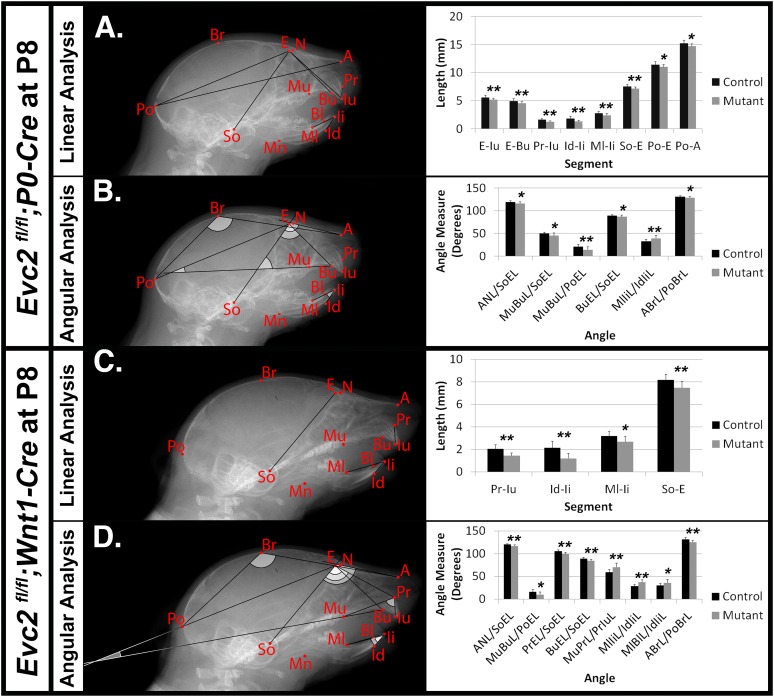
Differential mid-facial defects between *Evc2 P0* mutants and *Evc2 Wnt1* mutants at P8. Lateral X-ray cephalogram indicates the linear (A) and angular (B) measurements with significant differences between controls and *Evc2 P0* mutants. Lateral X-ray cephalogram indicates the linear (C) and angular (D) measurements with significant differences between controls and *Evc2 Wnt1* mutants. Graphs indicate the linear and angular values of the measurement with significant differences (*N* = 7; ^∗^*p* < 0.05; and ^∗∗^*p* < 0.01).
